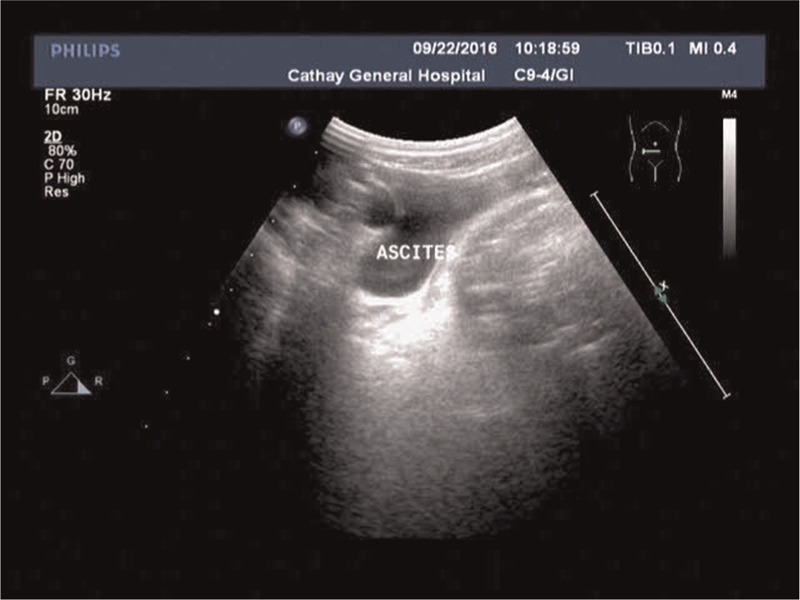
Ultrasound revealed ascites and a greatly dilated sigmoid colon.
